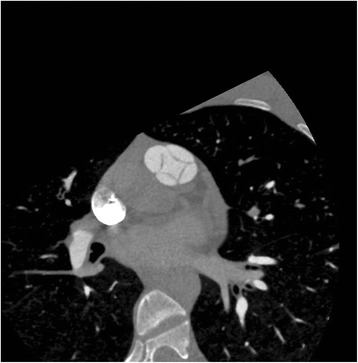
Low-dose prospectively gated multidetector computed tomography (MDCT) right ventriculogram, showing a QPV, a patent foramen ovale, and a normal right ventricular volume.
